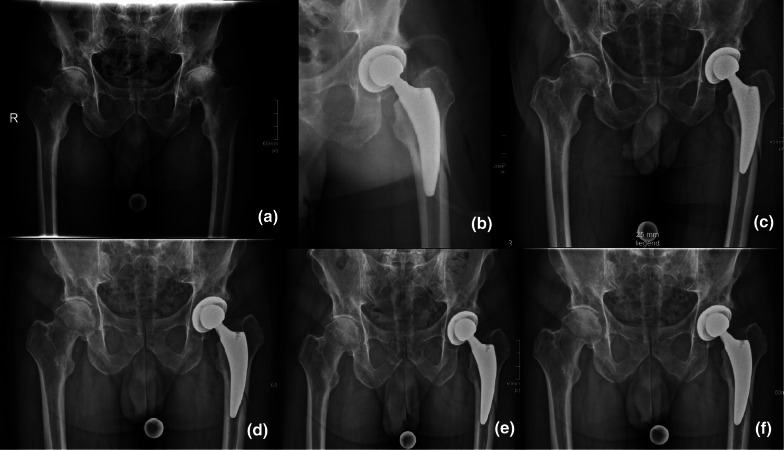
Intraoperative fracture treated without revision surgery; detected on day of surgery on postoperative x-ray with patient already outside the operating theater; patient did not give informed consent for revision surgery; conservative treatment with 6 weeks without weight-bearing; a preoperative, b postoperative, c discharge at home; d 6 weeks after surgery; e 3 months after surgery; f 1 year after surgery
